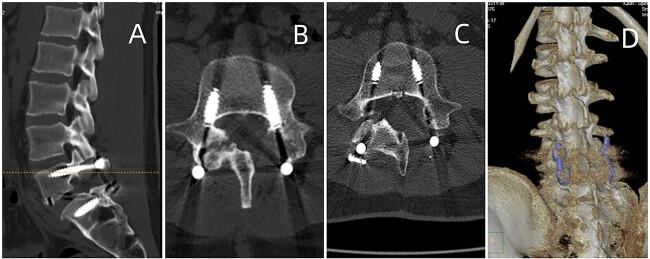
Postoperative CT of lumbar fusion surgery, depicted in (A) and (B). Two years postoperatively, DECT (displayed in (C) and (D)) reveals no urate deposition in the lumbar area.
